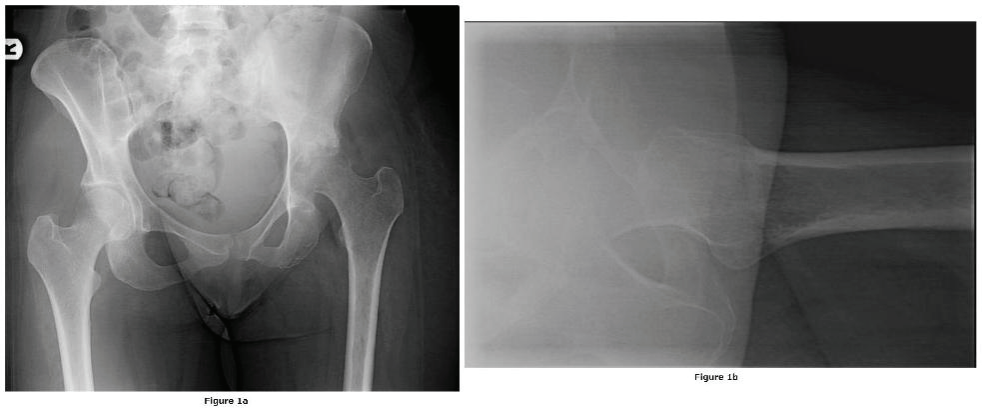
(a) Preoperative radiograph of the pelvis showing erosive destruction of the left hip joint with a superior migration of the femoral head indicating a significant acetabular bone loss. (b) Preoperative lateral radiograph of the left hip showing irregularities on the site of the femoral head and the same superior migration
